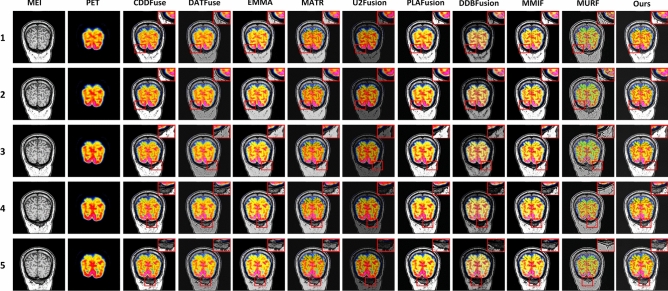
The comparison experiments fusion results of MRI brain images and PET images. Method 1: CDDFuse; Method 2: DATFuse; Method 3: EMMA; Method 4: MATR; Method 5: U2Fusion; Method 6: PLAFusion; Method 7: DDBFusion; Method 8: MMIF; Method 9: MURF; Method 10: Ours.
